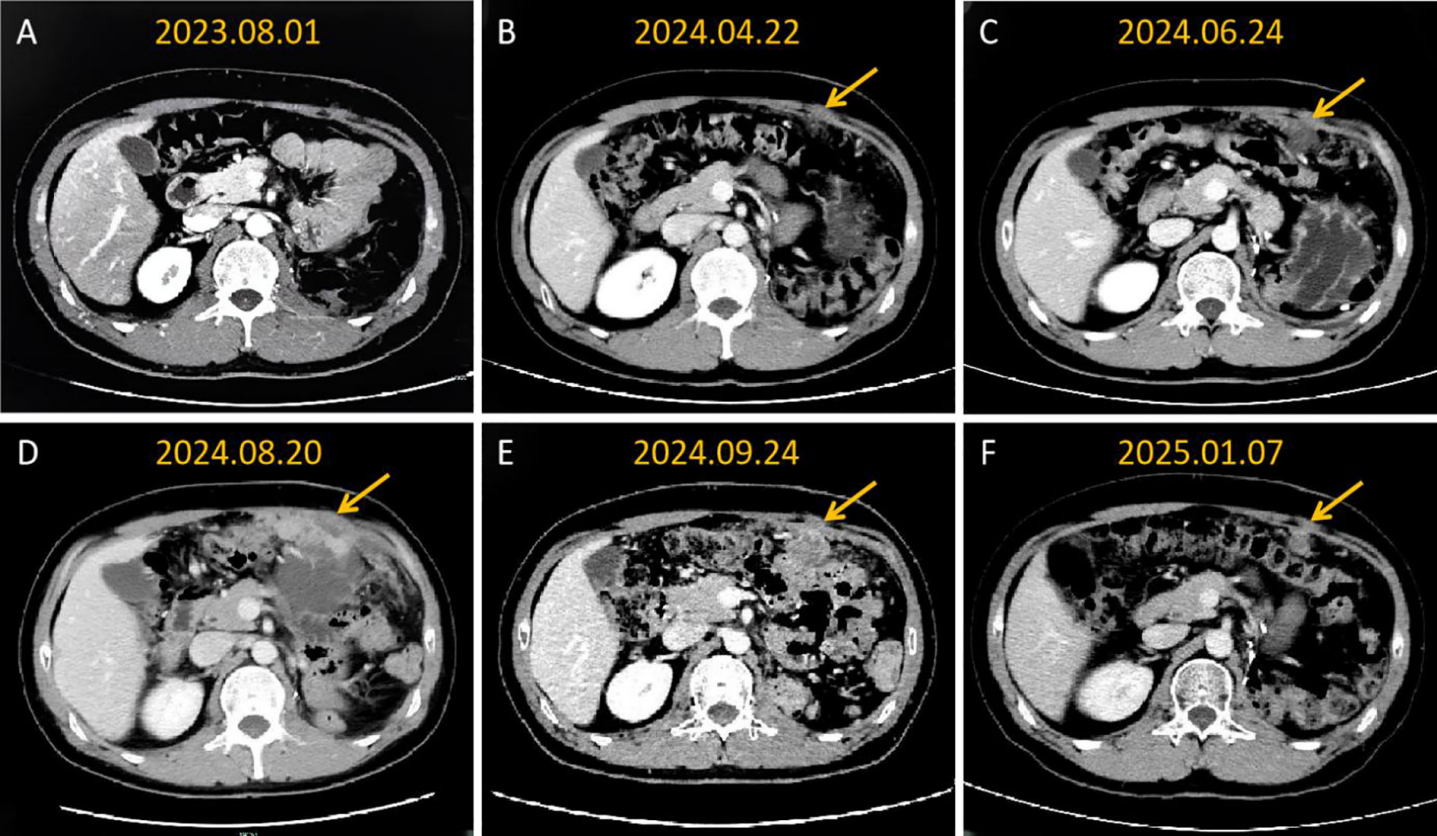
Contrast-enhanced CT imaging follow-up of abdominal wall metastatic lesions confirms sustained partial remission with sequential targeted therapy with abdominal artery infusion of bevacizumab. (A) The abdominal cavity shows postoperative changes, with no metastatic lesions observed; (B) Local nodular thickening begins to appear on the left anterior abdominal wall, suggesting recurrence and metastasis; (C) The metastatic tumor on the left anterior abdominal wall has increased in size; (D) The abdominal wall lesions have significantly enlarged, and extensive diffuse implantation metastasis occurs in the abdominal cavity; (E) After one month of local treatment, the abdominal wall and greater omentum lesions have significantly shrunk; (F) The abdominal cavity lesions continue to shrink.
